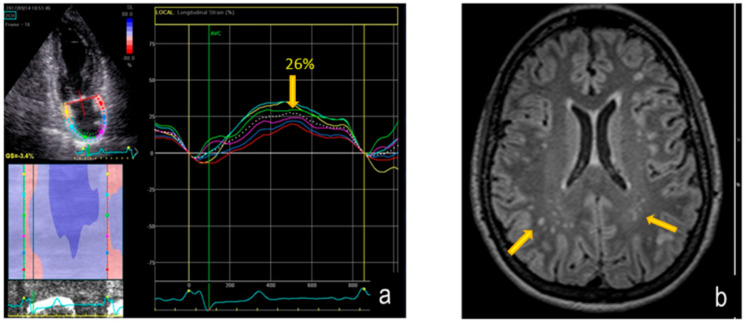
Reduction of PALS: upper left corner: two-dimensional echocardiography apical 2-chamber view, bottom left corner: color rendering of LA strain variation during cardiac cycle, right side: LA strain curves (a) and parallel axial FLAIR MRI image (b) showing multiple white matter hyper intensities lesions (arrows) in AFD patient. Fazekas score is = 1. Legend: PALS = peak atrial longitudinal strain; LA= left atrium; FLAIR = fluid attenuated inversion recovery; MRI = magnetic resonance imaging; AFD = Anderson-Fabry disease.
